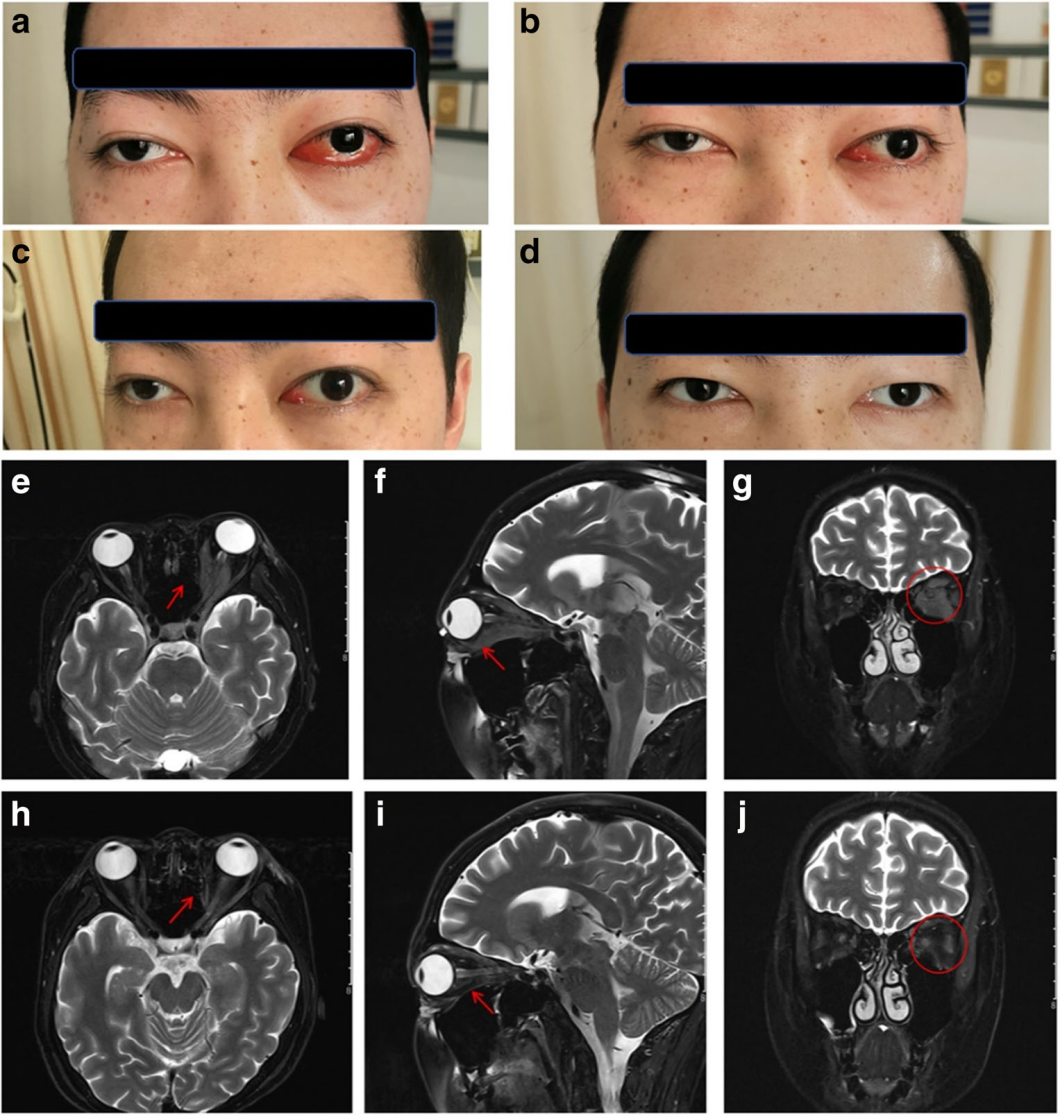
CD5-IL15/IL15sushi CAR T cells rapidly reduce patient’s orbital edema. a Prior to CD5-IL15/IL15sushi CAR treatment, the patient’s left eye had significant exophthalmos. Repeat imaging b 1 week after CD5-IL15/IL15sushi CAR infusion, c 2 weeks after infusion, d and 3 weeks following therapy demonstrate rapid resolution of the patient’s swelling. Prior to CD5-IL15/IL15sushi CAR treatment, MRI showed significant edema in the e axial, f sagittal, g and coronal planes. Red arrows and red circle mark the dense soft tissue infiltrate. 8 weeks following CD5-IL15/IL15sushi CAR treatment, repeat MRI imaging shows reduction in the soft tissue mass shadow in the h axial, i sagittal, j and coronal planes
